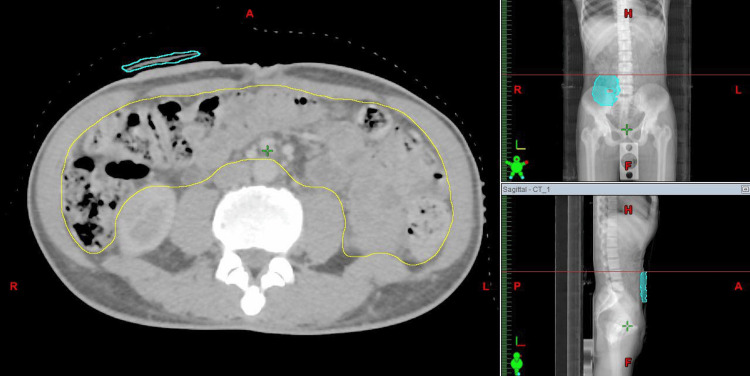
Representative axial computed tomography slice demonstrating the ostomy bag (blue) and bowel (yellow)
